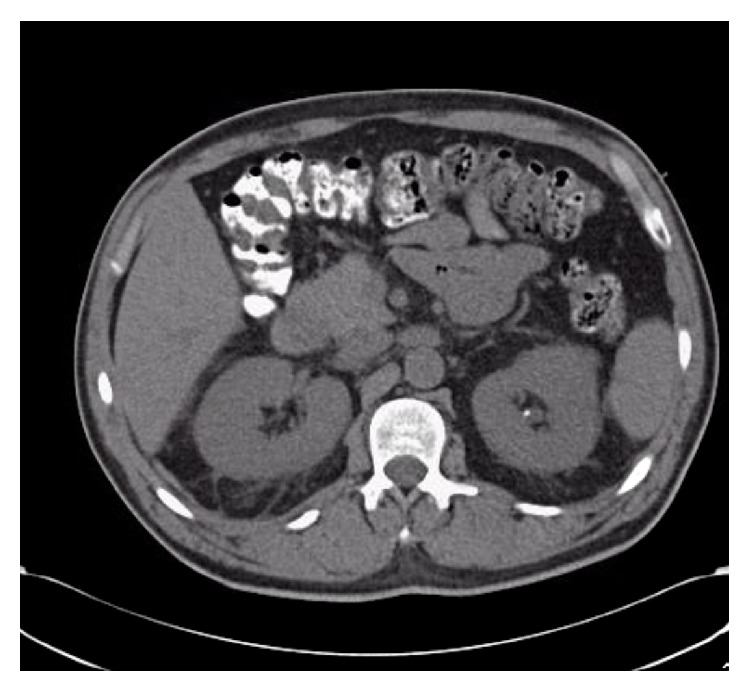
CT of abdomen and pelvis without IV contrast. CT of abdomen and pelvis without IV contrast showing a 3 mm nonobstructing left renal calculus with normal size kidneys and no nephrocalcinosis.
